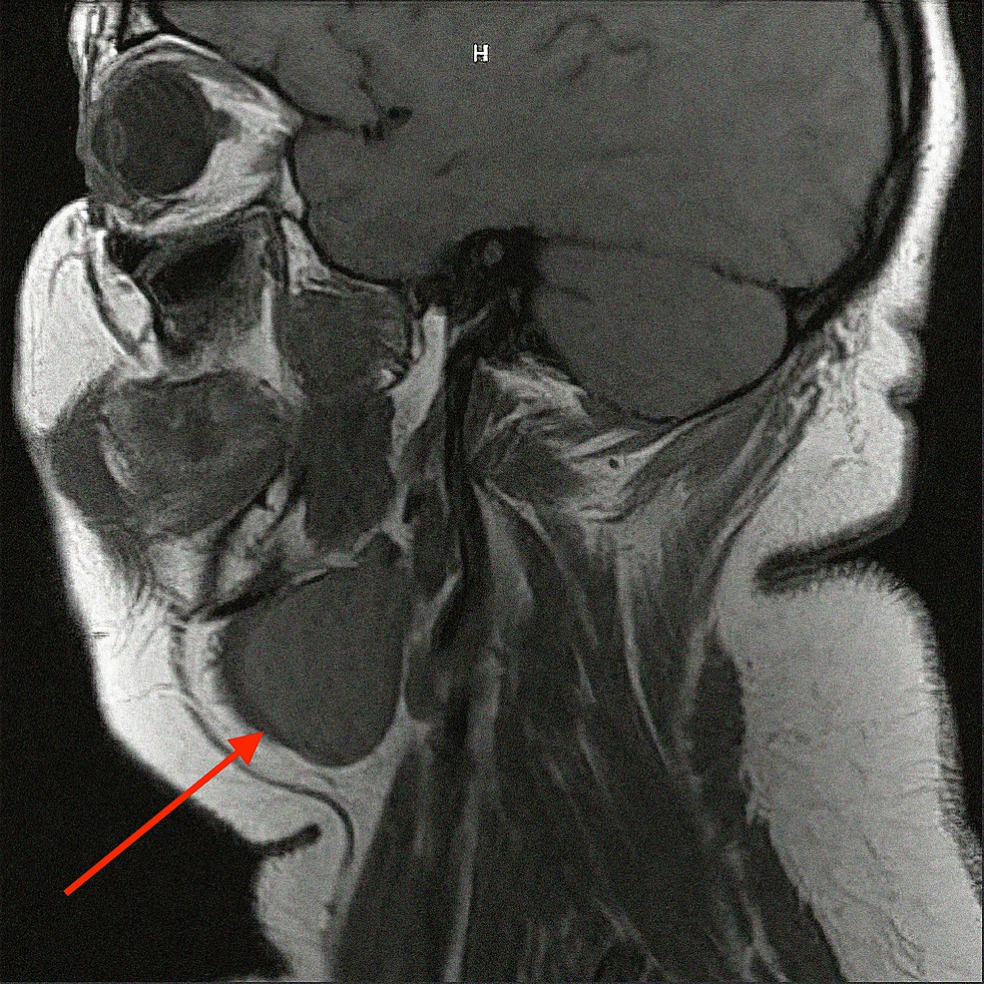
T1-weighted MRI of the head and neck with contrast, sagittal cut, demonstrating a thin-walled, nonenhancing, elongated cystic mass (red arrow) measuring 4.8 cm x 2.6 cm on the medial aspect of the left submandibular gland.
